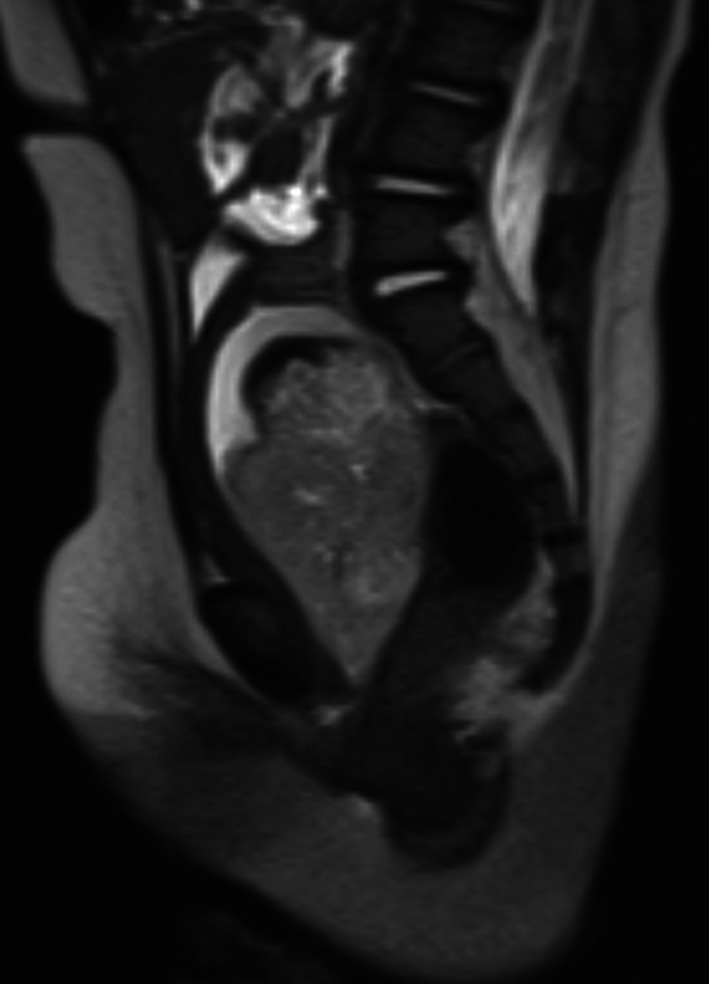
T2 sagittal image of the tumor at diagnosis. Magnetic resonance image shows a large solid mass in the vagina
